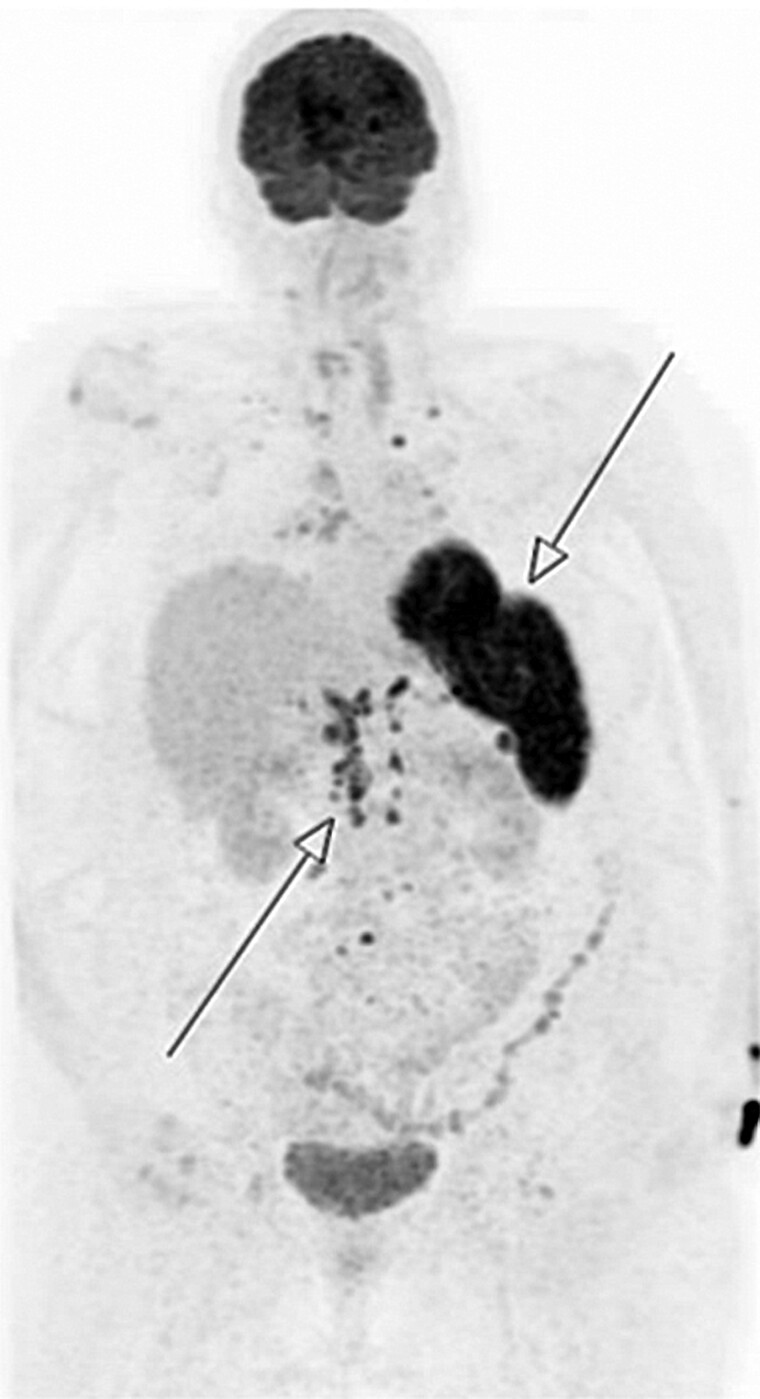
Positron emission tomography scan revealing fluorodeoxyglucose (FDG)-avid lymphadenopathy above and below the diaphragm coupled with FDG-avid uptake in the spleen.
